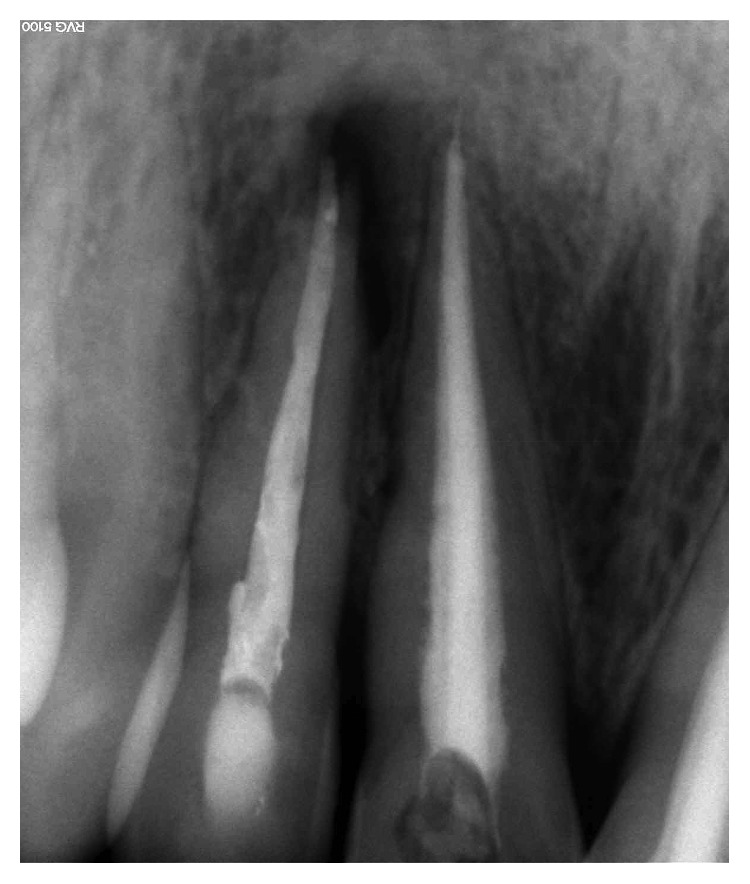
Radiograph of control 1 year later.
